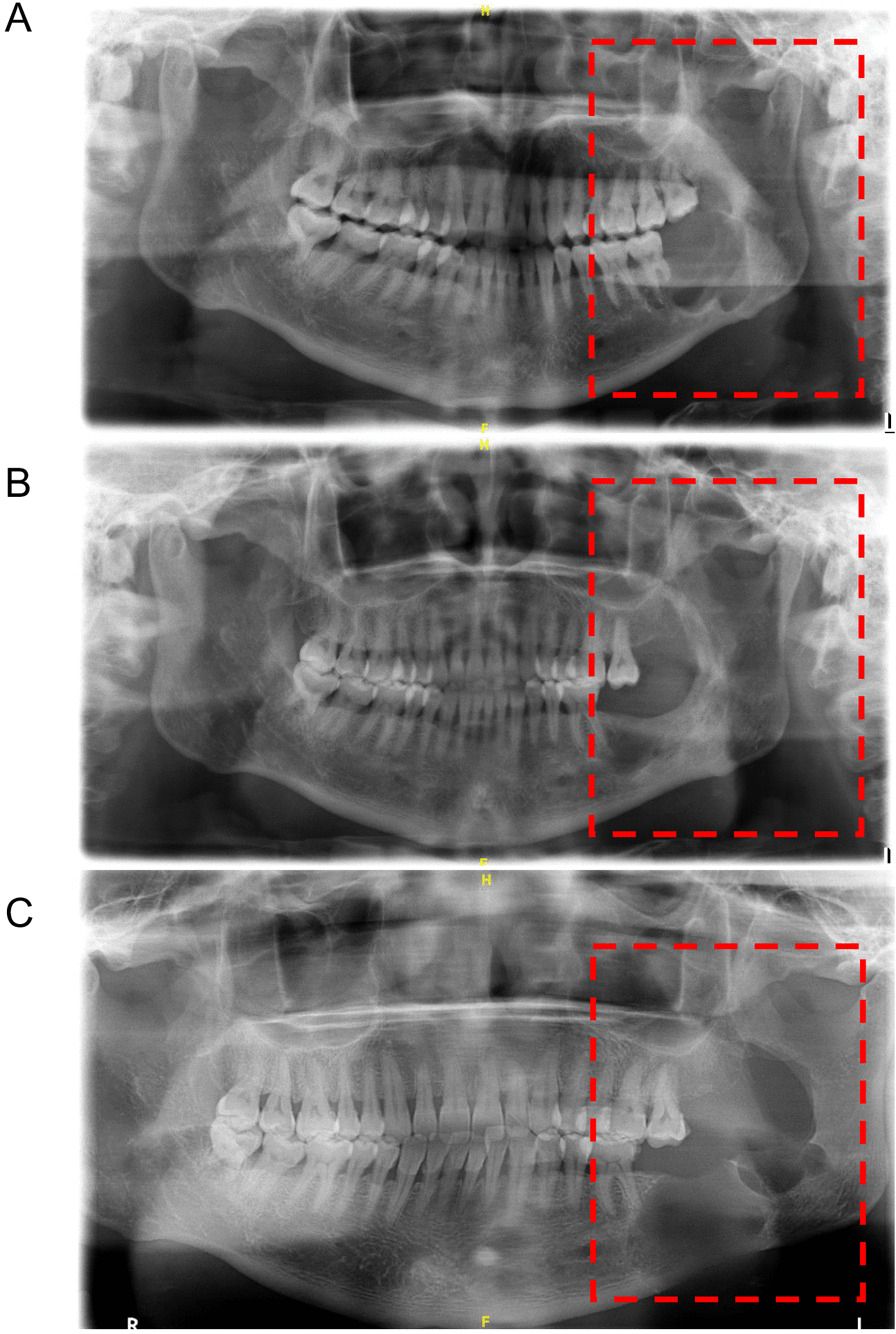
A case presentation of postoperative recurrence of MA. a Pre-operative panoramic radiography of patients; b The cystic cavity was reduced half a year after fenestration decompression; c Tumor recurrence 2 years after secondary curettage
